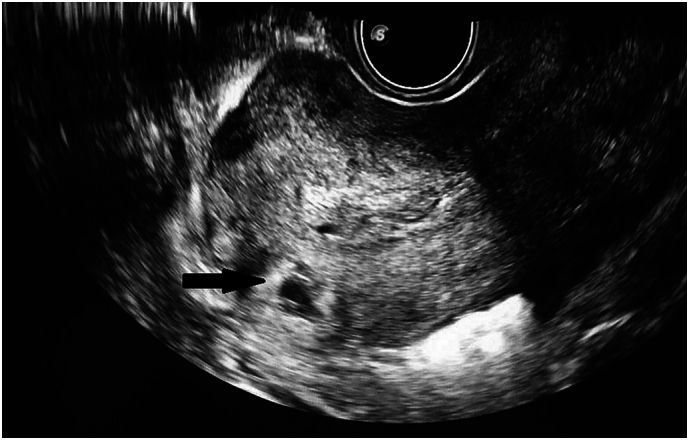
Toumi et al.'s figure 1 is a sonographic image of interstitial ectopic pregnancy (IEP).
